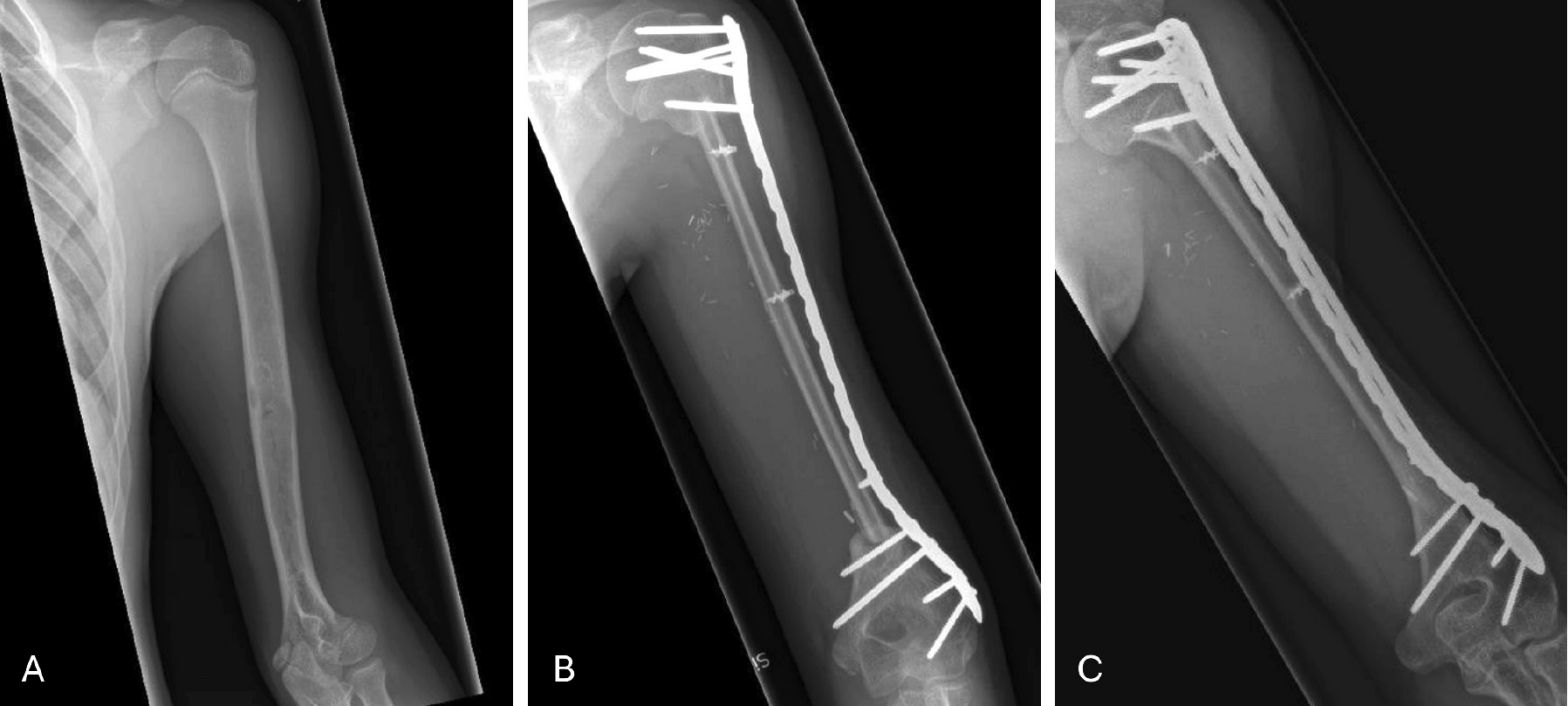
VFG following resection of osteosarcoma in the diaphysis of the humerus: (A) preoperative radiograph; (B) postoperative radiograph; (C) radiograph at 5 years postoperatively showing graft union and significant graft hypertrophy.
